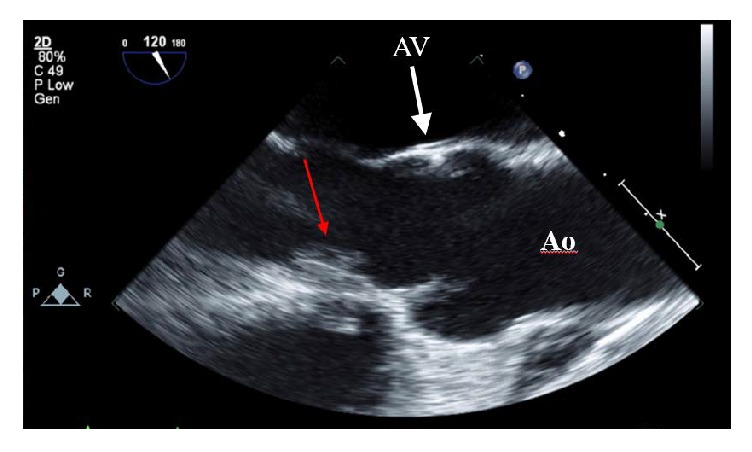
Transesophageal echocardiogram mid-esophageal position. The mass moves out of view during systole; red arrow indicates location of mass attachment to the left ventricular outflow tract. Ao = aorta and AV = aortic valve.
